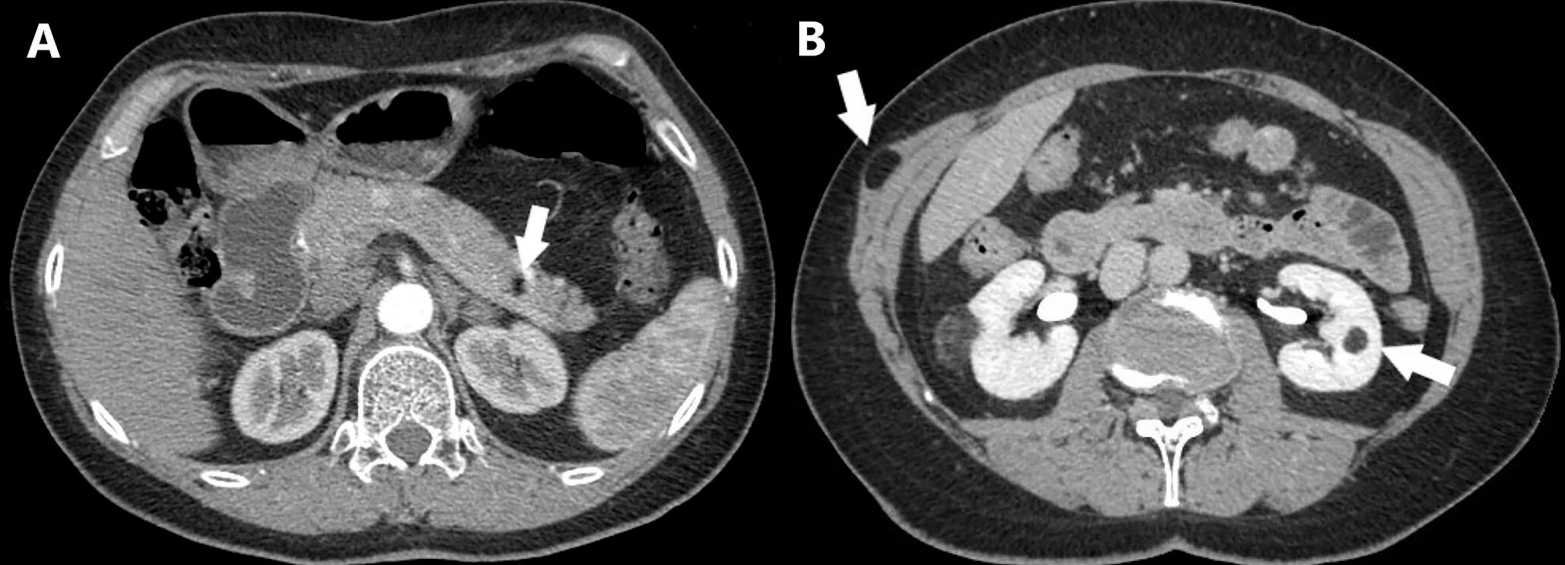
Axial CT scan of a MEN1 patient with multiple lipomatosis. (A) Intrapancreatic lipoma is shown (arrow). (B) Intramuscular lipoma and left renal angiomyolipoma are shown (arrows).
